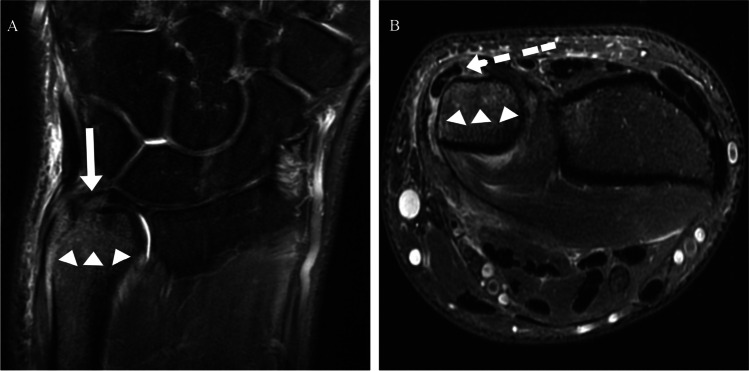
Coronal (A) and axial (B) T2-weighted fat-saturated sequences demonstrating a peripheral TFCC tear (white arrow) with ECU tendon subluxation and tenosynovitis (dashed white arrow), and distinct ulnar styloid BME (white arrowheads)
